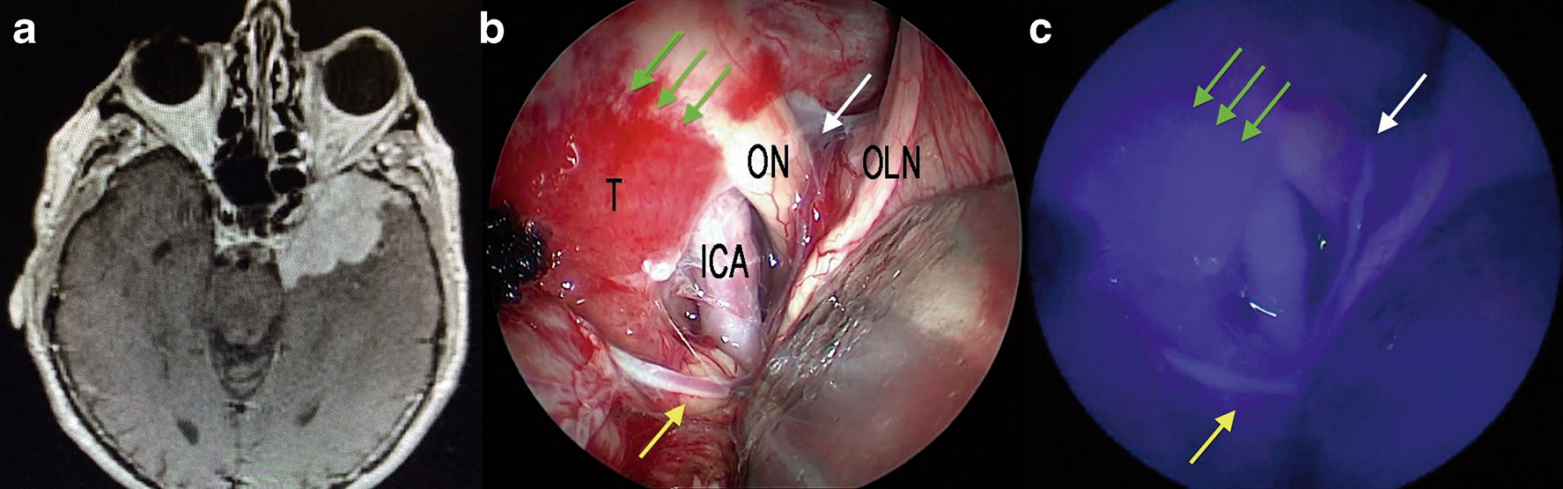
Intraoperative findings during spheno-orbital meningioma removal via a left pterional approach. Post-contrast T1-weighted brain MRI, axial view, showing the spheno-orbital meningioma extension. b Endoscopic assisted view showing the subfrontal exposure if the tumor under white light (a) and near-infrared light (c) after injection of ICG; *yellow arrow* shows a branch of the middle cerebral artery while the *green arrows* show the dural attachment of the lesion; the *white arrow* shows the a perforating artery over the left optic nerve
